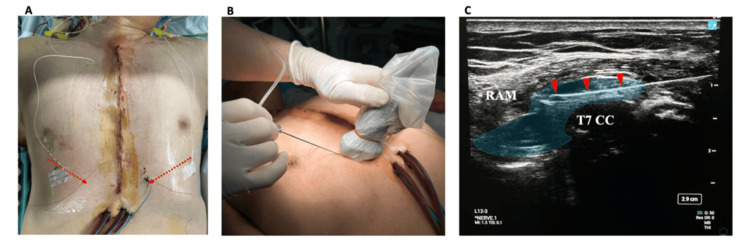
Rectointercostal Fascial Plane Block (RIFPB) and catheter placement. (A) The red dotted arrows indicate the direction of catheter placement. (B) Probe placement along the costal margin near the sternum, with the probe oriented diagonally from the nipple toward the epigastric region at the T7 level. (C) Ultrasound image showing local anesthetic (LA) spread beneath the rectus abdominis muscle (RAM) at the T7 costal cartilage (CC) level. The red arrowheads indicate the needle trajectory.
